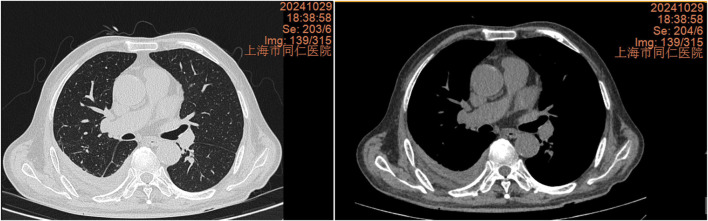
Chest CT scan 2 weeks after discharge on 29 October 2024, showing a significant reduction in right pleural effusion.
